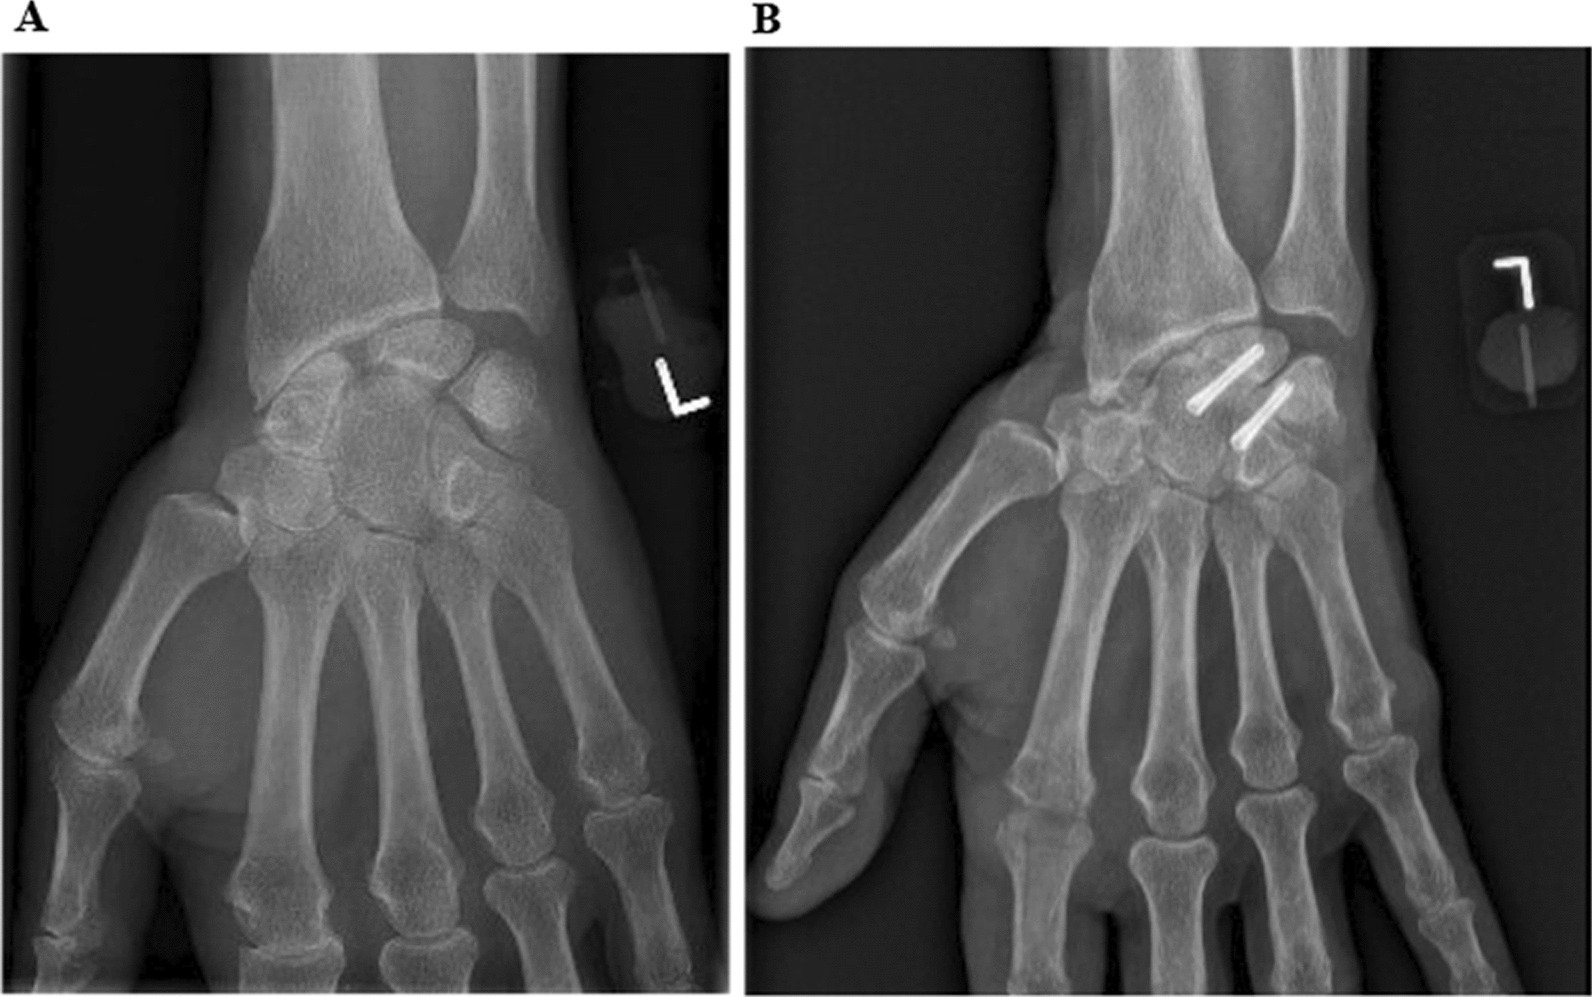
A 63-year-old male presenting a painful SLAC wrist. Preoperative X-rays (P-A plane) are showing signs of SLAC wrist. B Postoperative X-ray (P-A plane) showing no signs of osteoarthritic changes, 4 years after the 2CF surgery of the left wrist. The patient is pain-free
